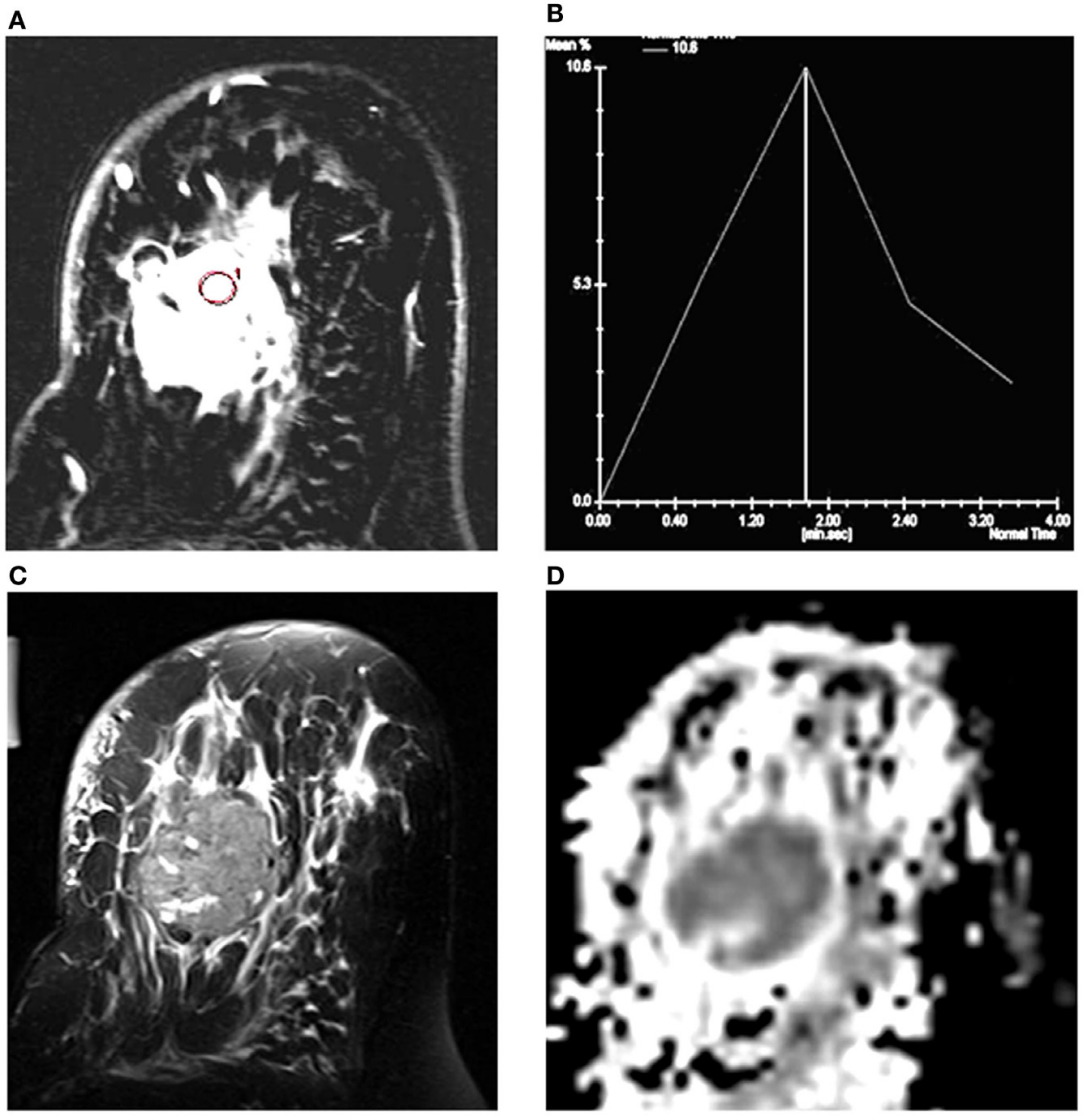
(A) T1-axial DCEMR image of a 56-year-old patient with infiltrating ductal carcinoma (IDC); (B) Type III dynamic curve with washout pattern from the ROI shown in the lesion; (C) T2-weighted fat-saturated axial image; and (D) the ADC map of the same patient.
